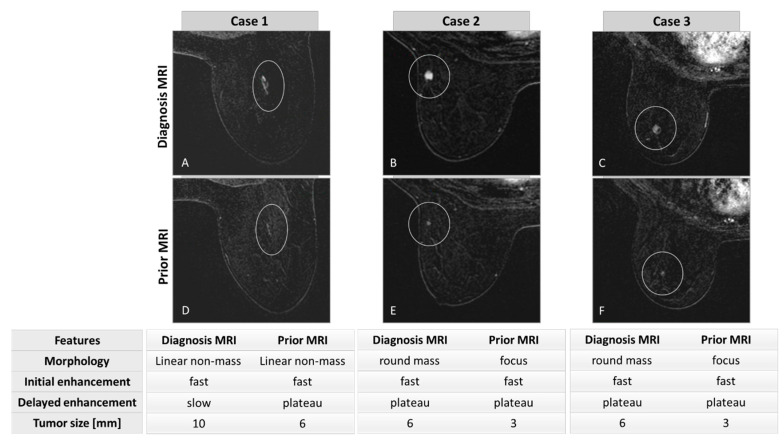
Diagnosed BCs in three patients (top row) and their corresponding radiological abnormalities as appearing in the previous MRIs (bottom row). Tumors and abnormalities are marked by a white circle. The morphological and kinetic features are shown below the images. Case 1–74 years old *BRCA1* PV carrier, diagnosed with grade 3 triple negative ductal carcinoma in situ (DCIS) (A). Linear non mass enhancement at the prior MRI (D) was unchanged throughout several previous MRIs (not presented), and therefore reported as BI-RADS 2. Case 2–64 years old *BRCA1* PV carrier, diagnosed with grade 3 triple negative invasive ductal carcinoma (IDC) (B). Enhancing focus at the prior MRI was misinterpreted as intramammary lymph node and reported as BI-RADS 2 (E). Case 3–67 years old *BRCA1* PV carrier, diagnosed with grade 3 triple negative IDC (C). Enhancing focus at the prior MRI was not detected by the radiologist and reported as BI-RADS 2 (F).
